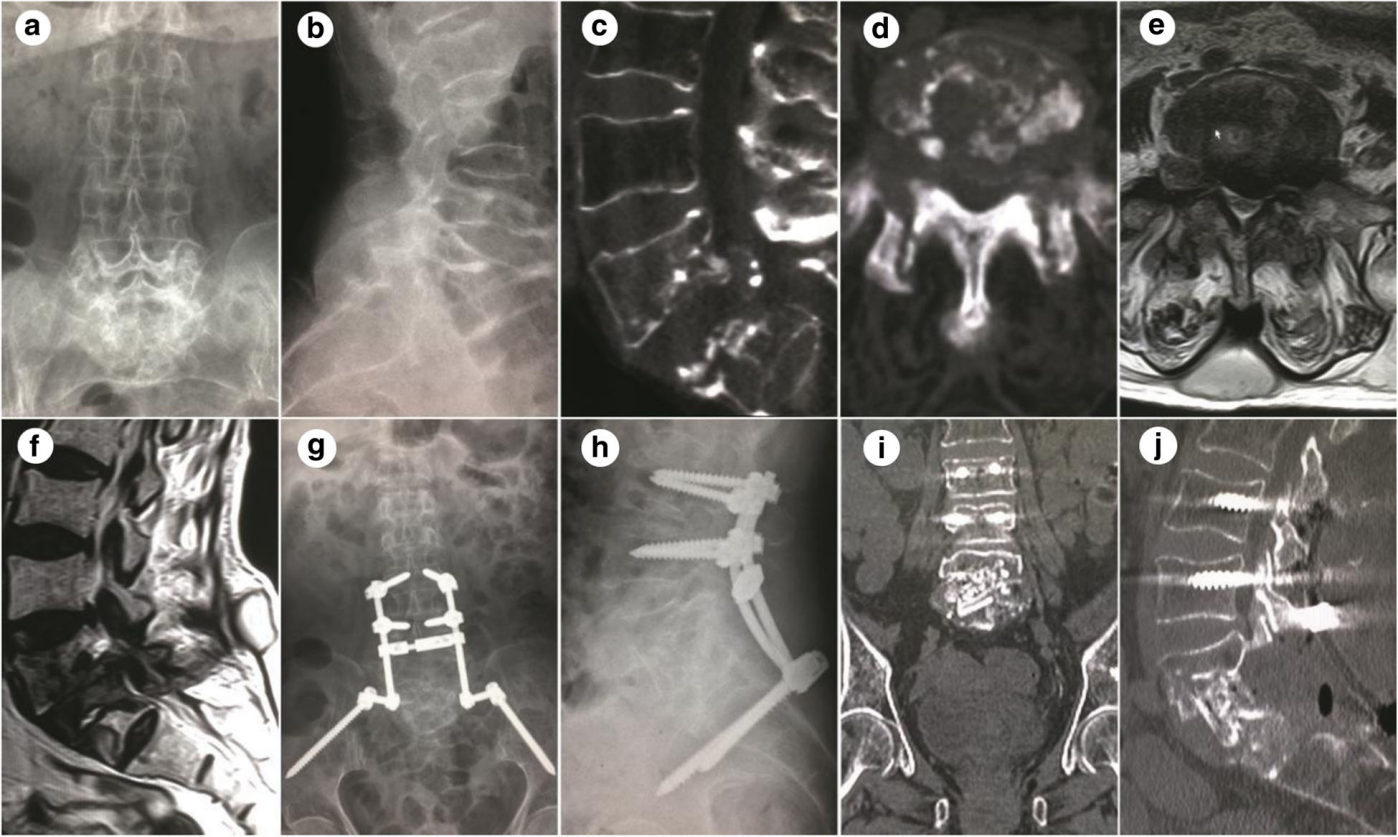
A 61-year-old female patient with lumbosacral tuberculosis with L5–S1 intervertebral lesion who also underwent posterior intervertebral space debridement with annular bone graft fusion and fixation. a-f Preoperative images. Preoperative computed tomography (CT) and magnetic resonance imaging showed bone destruction and extensive flow abscess (c–f). g-j Postoperative radiographs. Postoperative CT in the coronal and sagittal planes at 18 months showed a good position of internal fixation and excellent intervertebral bone fusion
